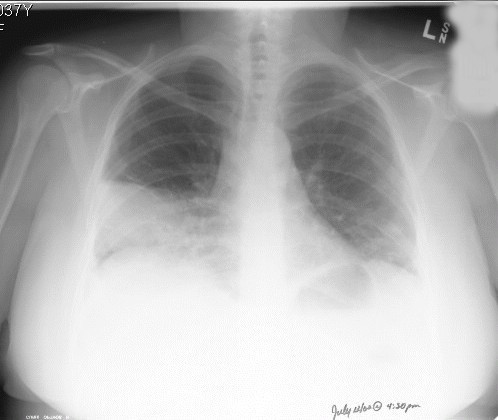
Chest X-ray (PA view), 5 days later, showing progression of disease with infiltrates in right and left lower lobes.
